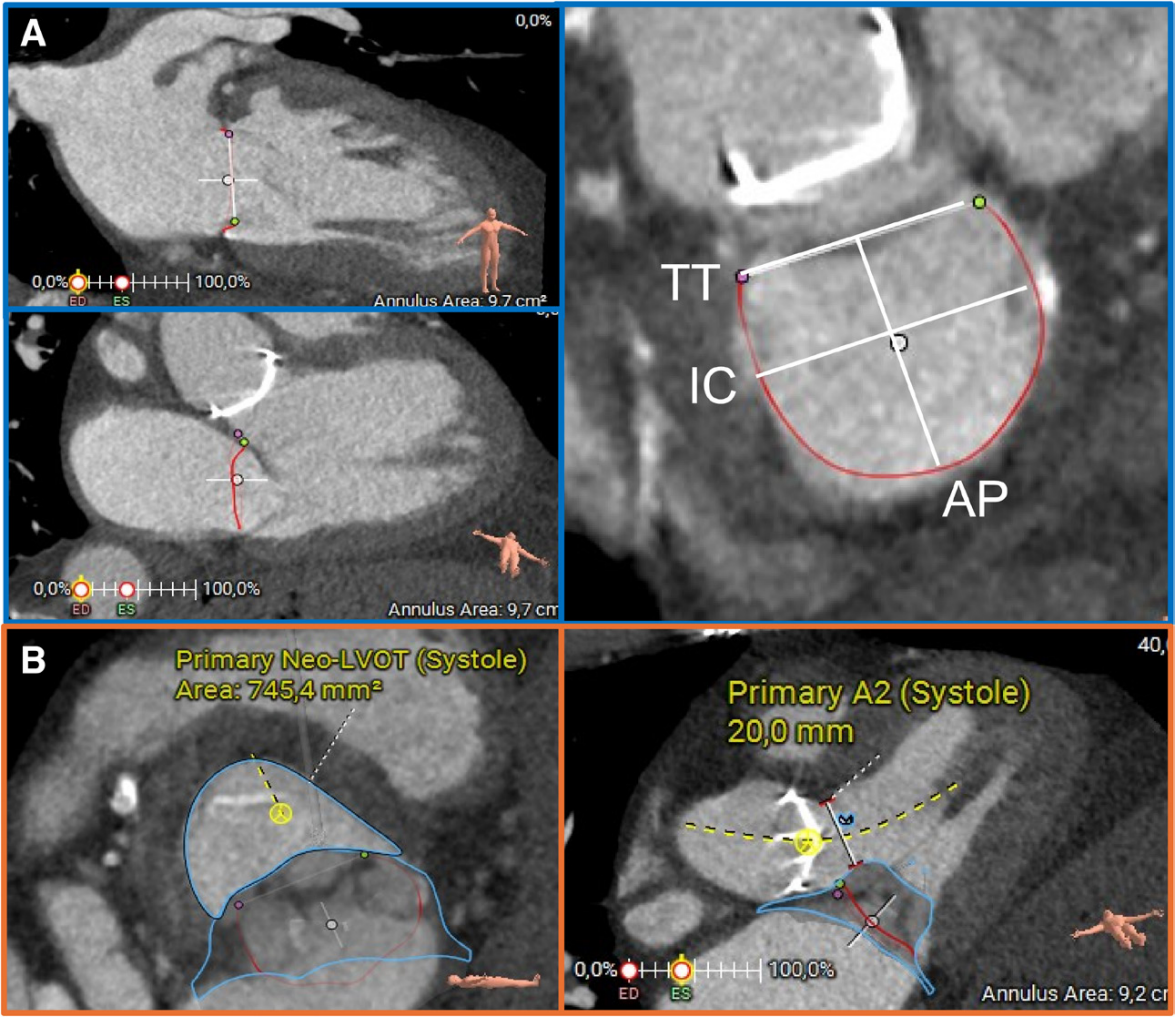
Cardiac computed tomography showing measurement of mitral annulus and neo–left ventricular outflow tract (LVOT). Screening for Tendyne in a patient with previous surgical aortic valve replacement and myectomy. A, D-shaped annulus with relevant measurements in 2-chamber view (upper left), 3-chamber view (lower left), and short axis (right): trigone-to-trigone (TT) distance, intercommissural (IC) distance, and antero-posterior (AP) distance. B, Neo-LVOT area (left) and diameter (right).
